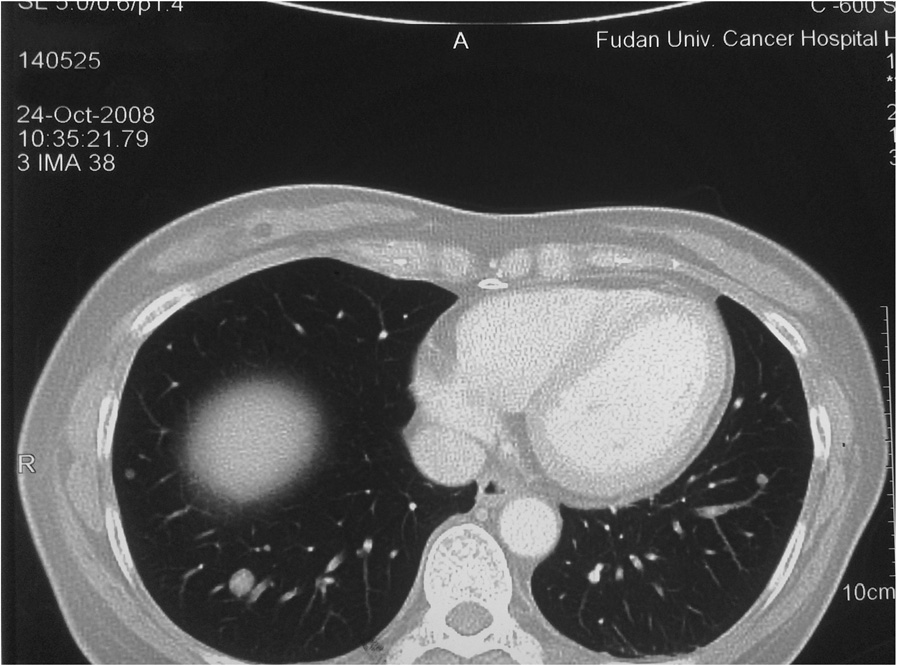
CT of Case 2 shows multiple nodules in both lungs.
